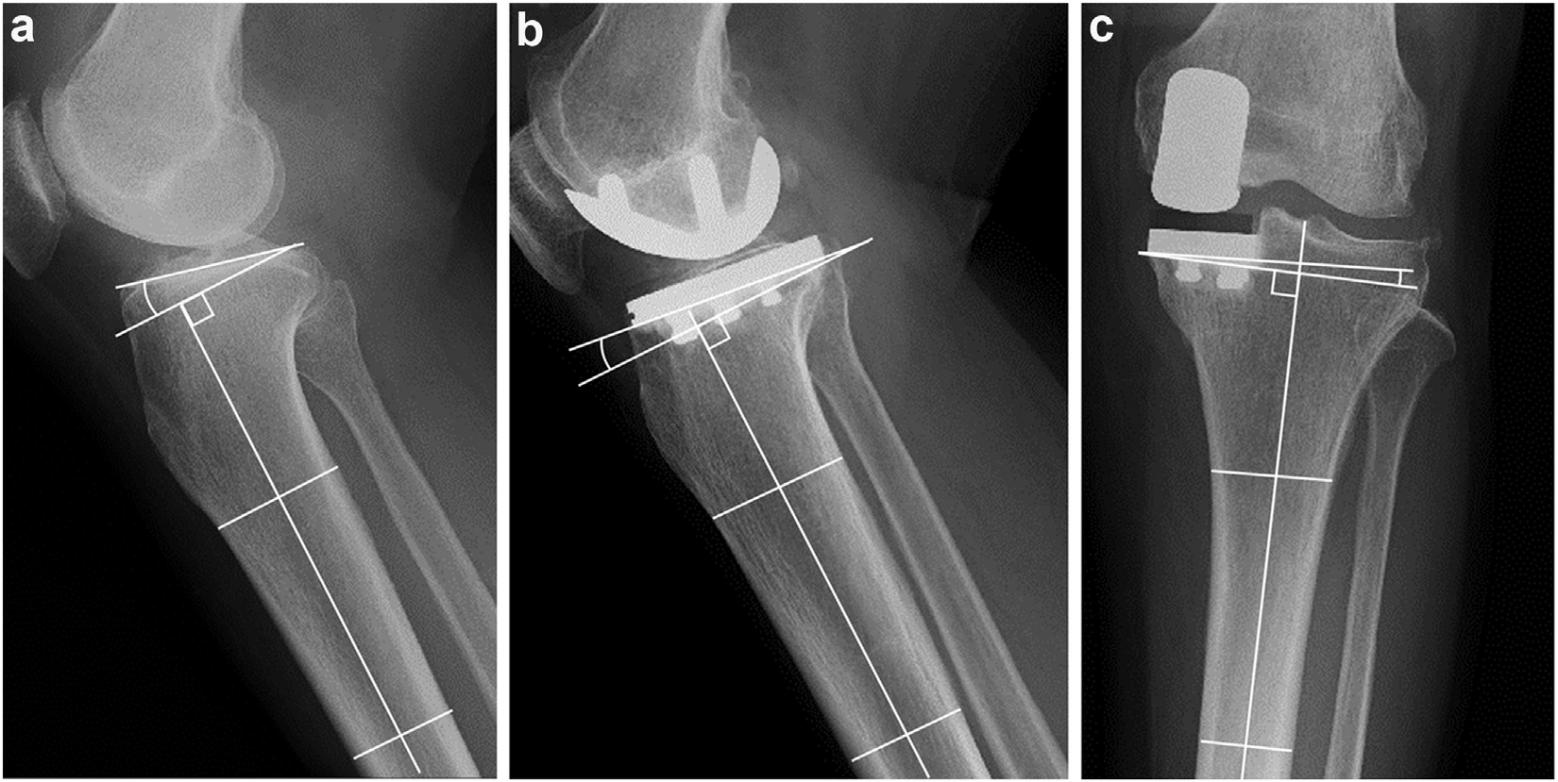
Radiographic measurement. Preoperative (a) and postoperative (b) posterior tibial slope and postoperative varus alignment of tibial component placement (c) were measured.
